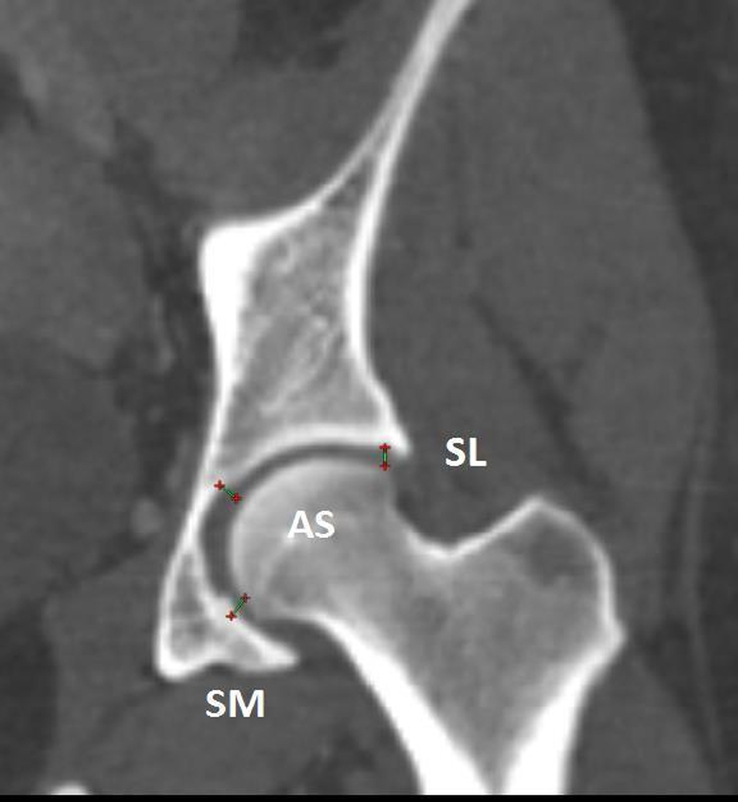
Reformatted coronal computed tomography image of left hip joint showing method for measurement of joint space width. AS, joint space width at apical site; SL, joint space width at superolateral site; SM, joint space width at superomedial site.
